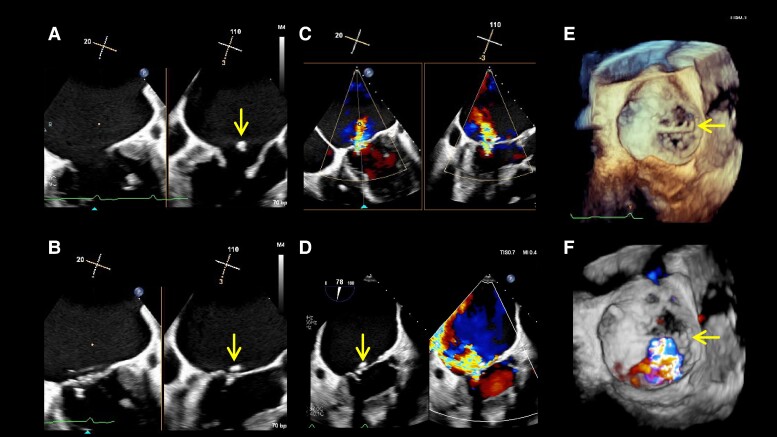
Multiplane view presenting the commissural view (left: 20°) and left ventricular out tract view (right: 110°) in transoesophageal echocardiography (TOE) (*A*: end-diastole, *B*: end-systole, and arrows: detached annuloplasty ring). Detached annuloplasty ring and atrial functional mitral regurgitation (*C* and *D*) and its three-dimensional TOE image (*E* and *F*).
